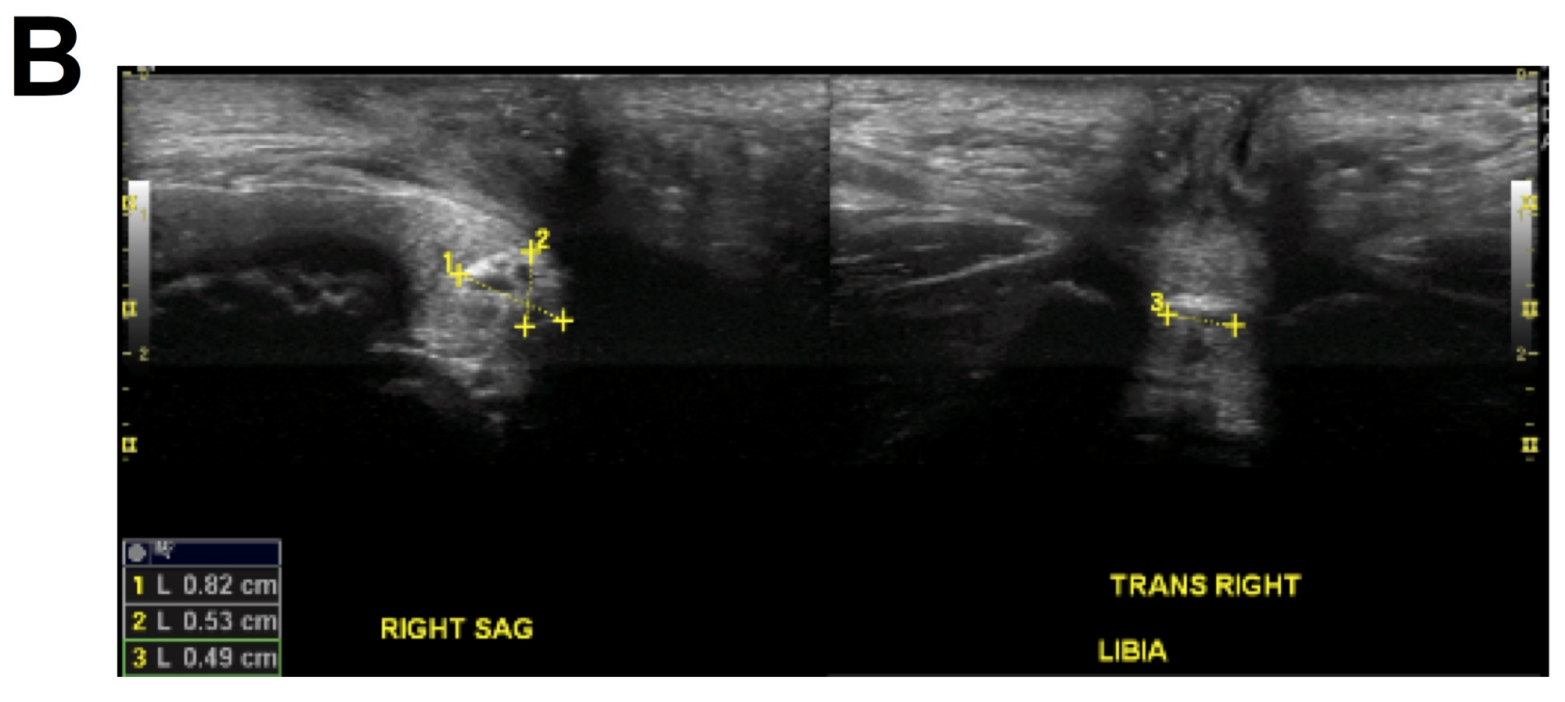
Ultrasound scan of the pelvis showed an echogenic structure measuring 0.8 cm x 0.5 cm x 0.5 cm in the region of the vagina with areas of venous flow
